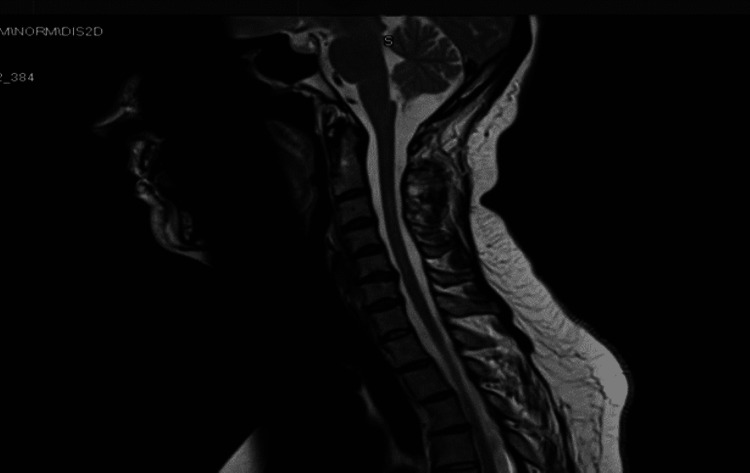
MRI of the cervical spine showing the reduction in T2 hyperintensity of intramedullary abnormal signal intensity extending from C2 to C7 level MRI: Magnetic resonance imaging.
